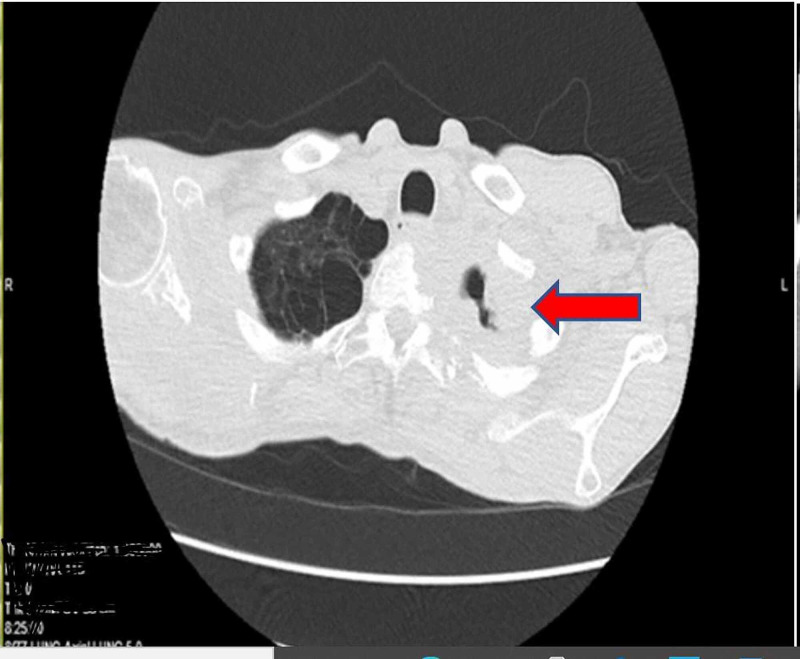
Axial section CT chest without contrast. Red arrow pointing to apical mass.
